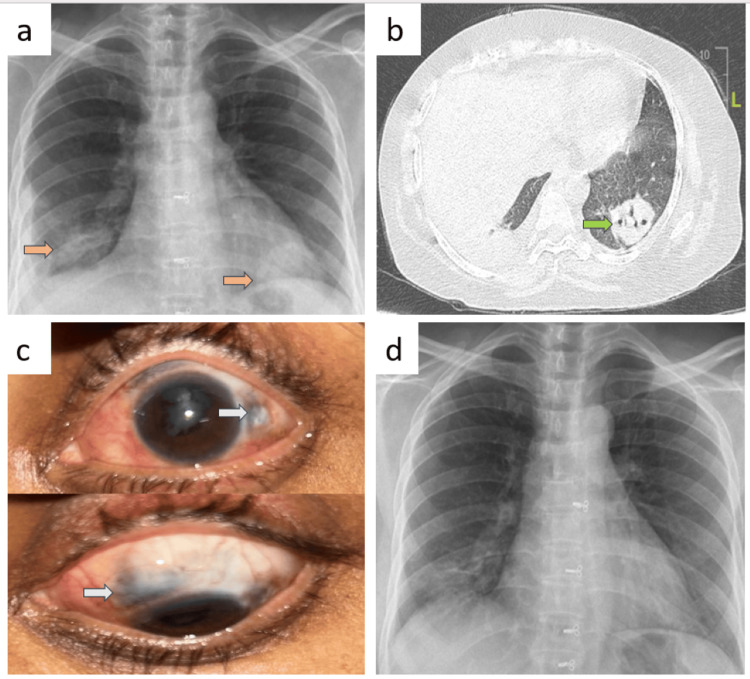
Radiology and ophthalmology images of patient B before and after treatment. (a) Chest X-ray showed a non-homogenous opacity in the right lower zone with costophrenic angle blunting and left lower zone rounded opacity marked by brown arrows. (b) CT showed cavitating consolidation in the left lower lobe marked by a green arrow. (c) The left eye had scleritis and scleral necrosis marked by white arrows. (d) Follow-up chest X-ray showed near-complete resolution.
